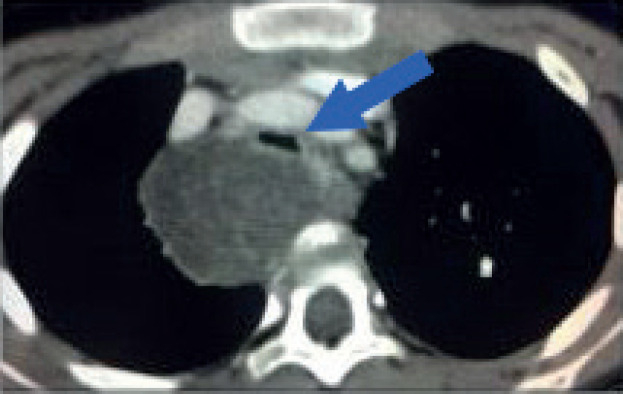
Tracheal compression. Contrast-enhanced axial CT image of a 6-year-old girl with a large tumour at the upper right posterior mediastinum. The lesion crosses the midline over the anterior surface of the vertebral body, at the level of the manubrium. Both, arterial and venous mediastinal great vessels are selectively enhanced with contrast. The trachea is severely compressed by the tumour (Blue arrow). This patient is at high risk of ventilatory collapse during the induction of anaesthesia.
